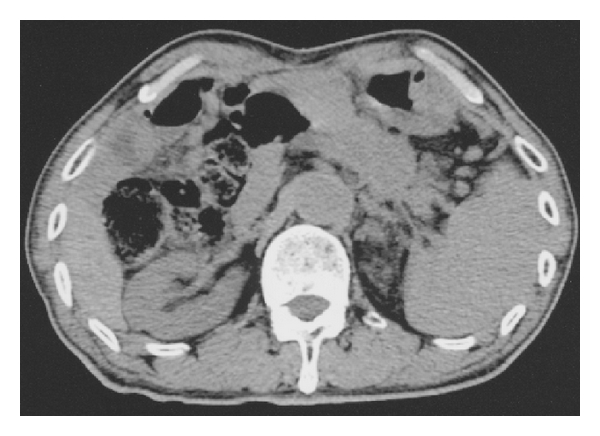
Case 1: A noncontrast CT 137 months after surgical treatment demonstrating enlargement of the remnant pancreas.
